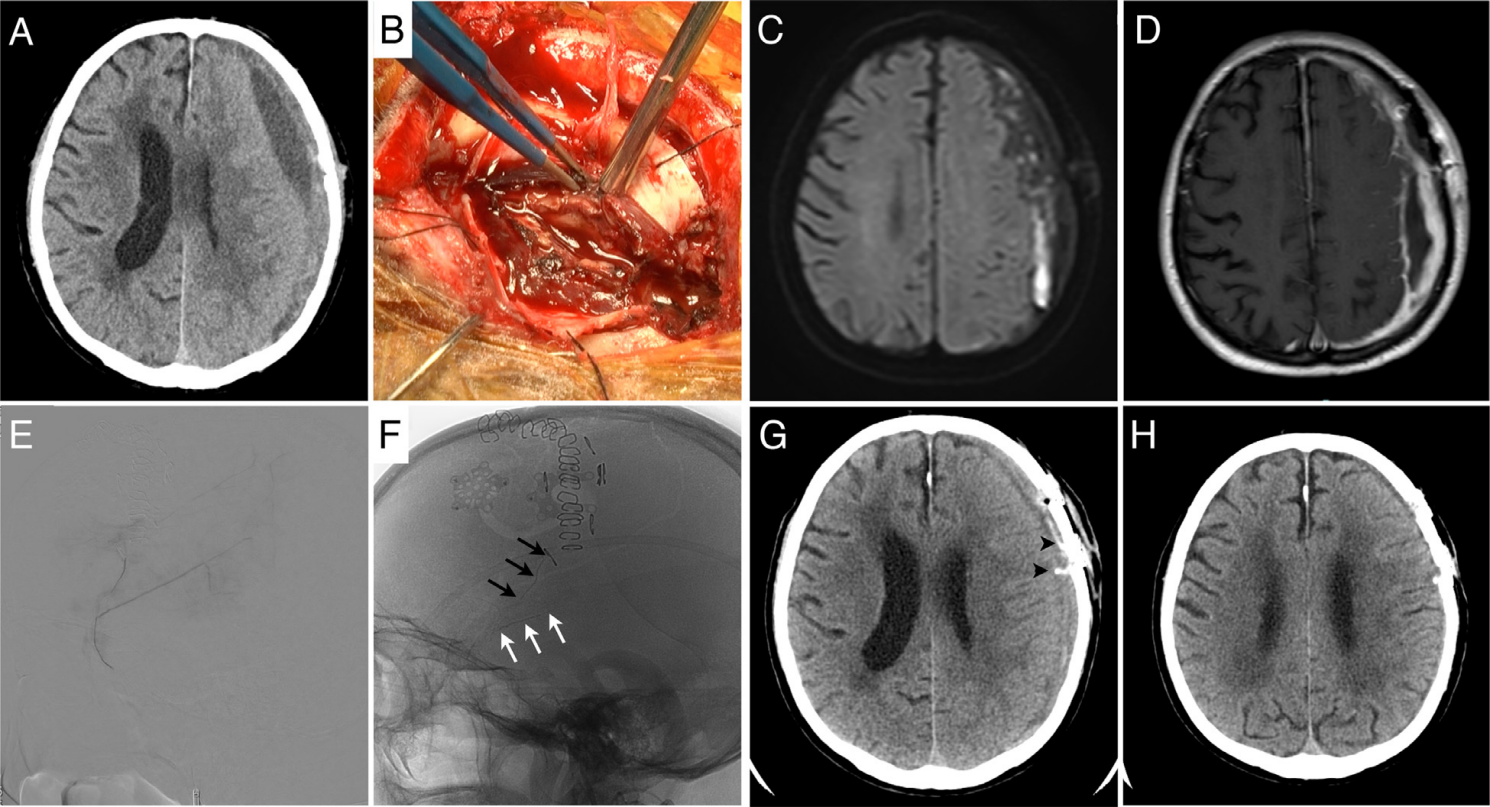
An 83-year-old male with a history of left chronic subdural hematoma drainage presented with right hemiplegia. (A) A head computed tomography (CT) scan on admission showed a mixed-density left subdural hematoma with midline shift. (B) A small craniotomy and dura incision revealed a solid hematoma, and the outer thick membrane was removed as much as possible. (C, D) Diffusion-weighted images and gadolinium-enhanced T1-weighted images showed the solid vascularized membrane of an organized chronic subdural hematoma (OCSDH). (E) A lateral view of an angiogram of the left middle meningeal artery showed the vascular foci feeding the hematoma. (F) The left middle meningeal artery embolization was performed with 25% n-butyl-2-cyanoacrylate (NBCA) for the frontal branch (black arrows) and parietal branch (white arrows). (G) A postoperative CT scan showed the injected NBCA in the inner and outer membrane of OCSDH (arrowhead). (H) A CT scan revealed that the hematoma had resolved, with no recurrence in 6 months.
